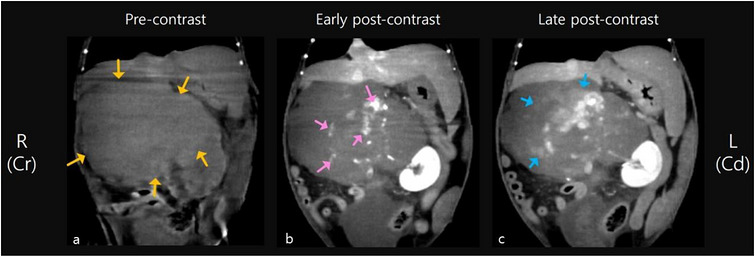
Contrast‐enhanced computed tomography (CT) of the abdomen at initial diagnosis, dorsal plane, soft‐tissue window. (a) Pre‐contrast image showing a large retroperitoneal mass (12.1 × 8.0 × 8.5 cm) of homogeneous soft‐tissue attenuation (yellow arrows). (b) Early post‐contrast image revealing heterogeneous enhancement with focal regions of strong contrast uptake (pink arrows). (c) Late post‐contrast image demonstrating progressive contrast distribution into surrounding parenchyma (blue arrows).
